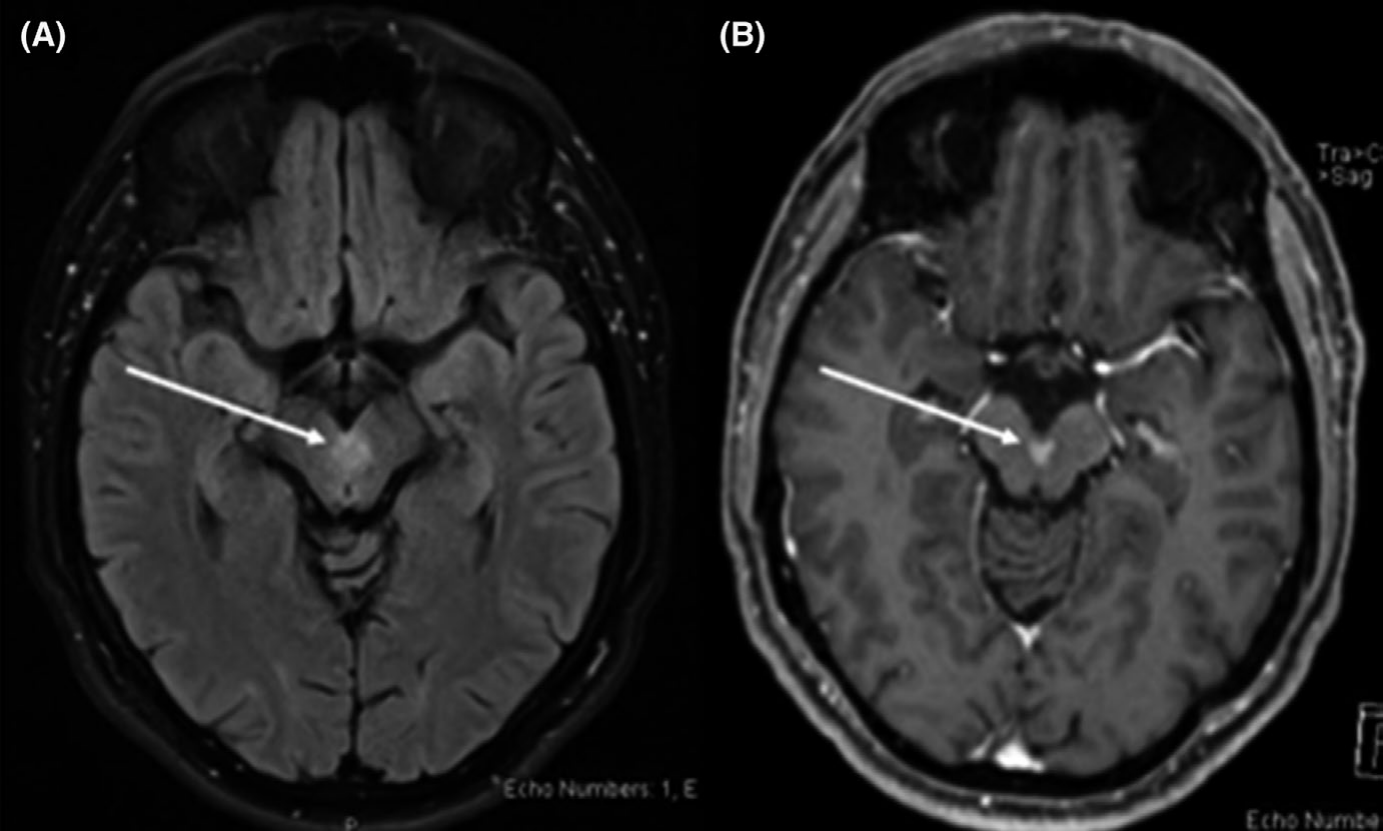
Three‐month magnetic resonance imaging (MRI) brain follow‐up, (A) axial FLAIR‐T2 (fluid attenuated inversion recovery), and (B) axial T1‐weighted (T1WI) post I.V. Gadolinium‐based contrast (Gd), demonstrates a midbrain midline lesion centered with interval regression with decreased T2 bright signal and decreased post‐contrast enhancement (arrow in A and B)
